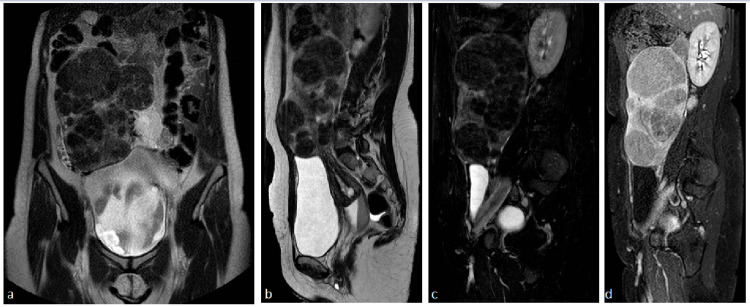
Preprocedural MRI images a) Coronal T2-weighted image shows an enlarged and elongated uterus body (222 mm x 163 mm), with numerous focal lesions demonstrating low signal. Deformation of the urine bladder can be observed. b) Sagittal T2-weighted image in the midline shows the cranial elongation of the uterine corpus, the cervix, and vagina. Nabothian cysts can be seen in the cervix. c) Sagittal T2-weighted fat-saturated image shows the maximum craniocaudal length (222 mm) of the uterus, which was measured laterally towards the right upper quadrant of the abdomen. d) Sagittal T1-weighted, contrast-enhanced, fat-saturated image shows enhancement of all the fibroids.
